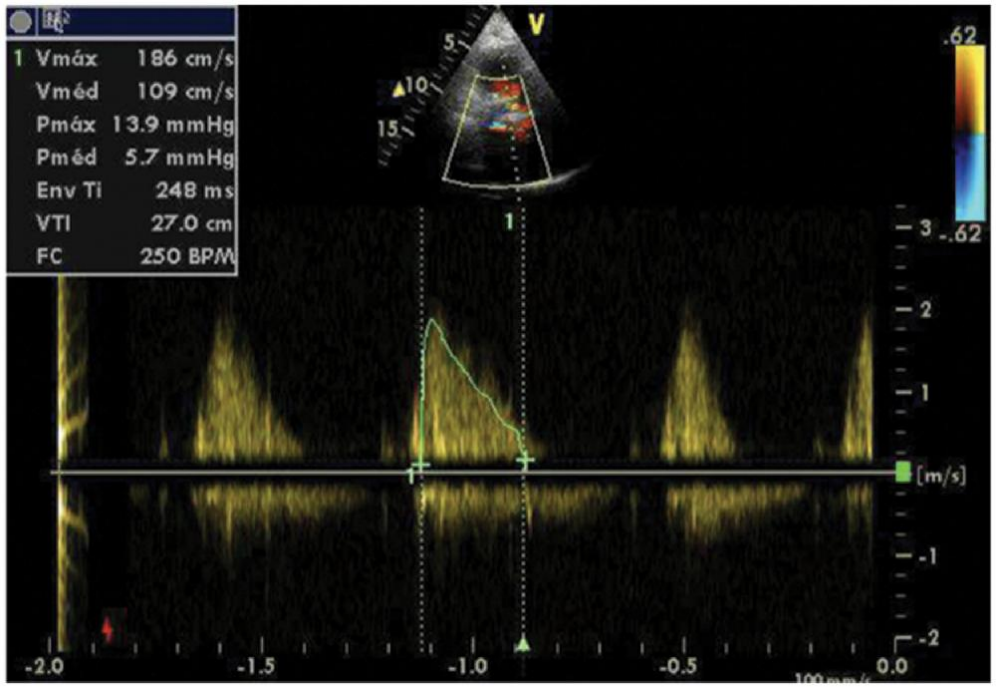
Post-procedural echocardiogram reveals a significant reduction in transprosthetic pressure gradient (from 12 to 5 mmHg) and a well-placed valve [91.5 × 133 mm (×DPI)].
